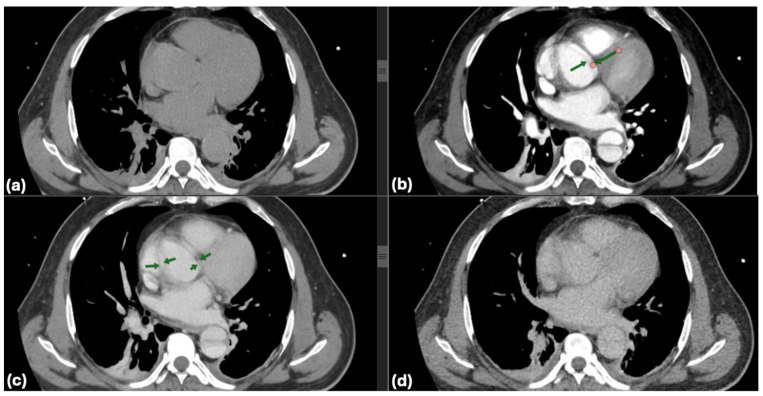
Artifacts in non-gated computed tomography: pulsation effects in aortic imaging: common artifacts from pulsation in non-gated computed tomography (CT) imaging are highlighted with green arrows (b,c). These artifacts can obscure critical details of the aortic wall and luminal structure, potentially complicating the assessment of aortic dissections (a,d). Accurate interpretation requires recognizing and mitigating such limitations.
